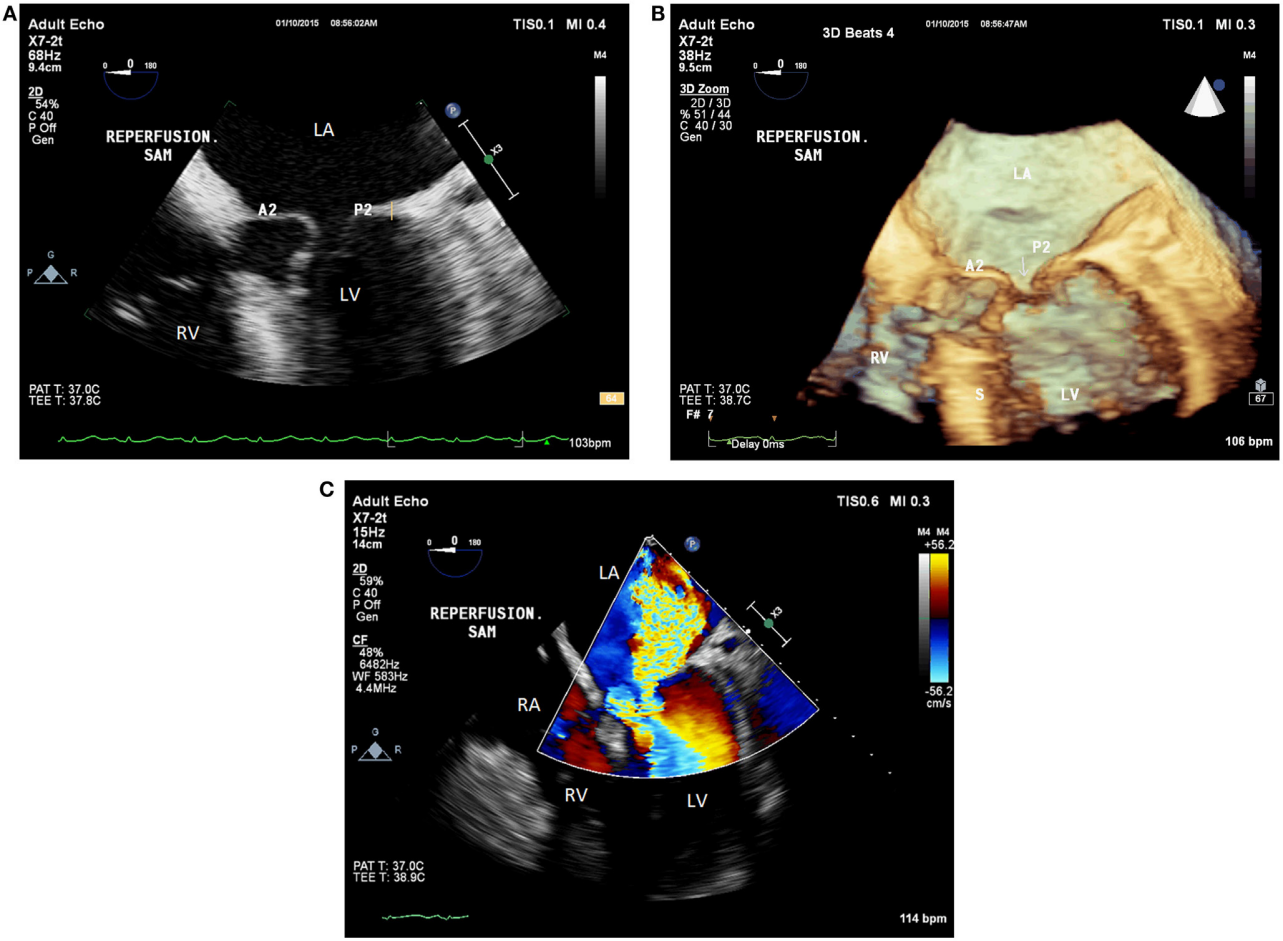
(A) Postreperfusion 2D TEE midesophageal modified five-chamber view demonstrating systolic anterior motion of the anterior mitral valve leaflet causing severe left ventricular outflow tract obstruction. (B) Postreperfusion 3D TEE midesophageal modified five-chamber view demonstrating left ventricular outflow tract obstruction resulting from systolic anterior motion of the anterior mitral valve leaflet. (C) Postreperfusion 2D TEE midesophageal modified five-chamber view with color flow Doppler, demonstrating severe posterolateral mitral regurgitation caused by systolic anterior motion of the anterior mitral valve leaflet. LV, left ventricle; RV, right ventricle; LA, left atrium; RA, right atrium; A2, second segment of the anterior mitral valve leaflet; P2, second segment of the posterior mitral valve leaflet; S, interventricular septum; Arrow, coaptation defect between the mitral leaflets.
